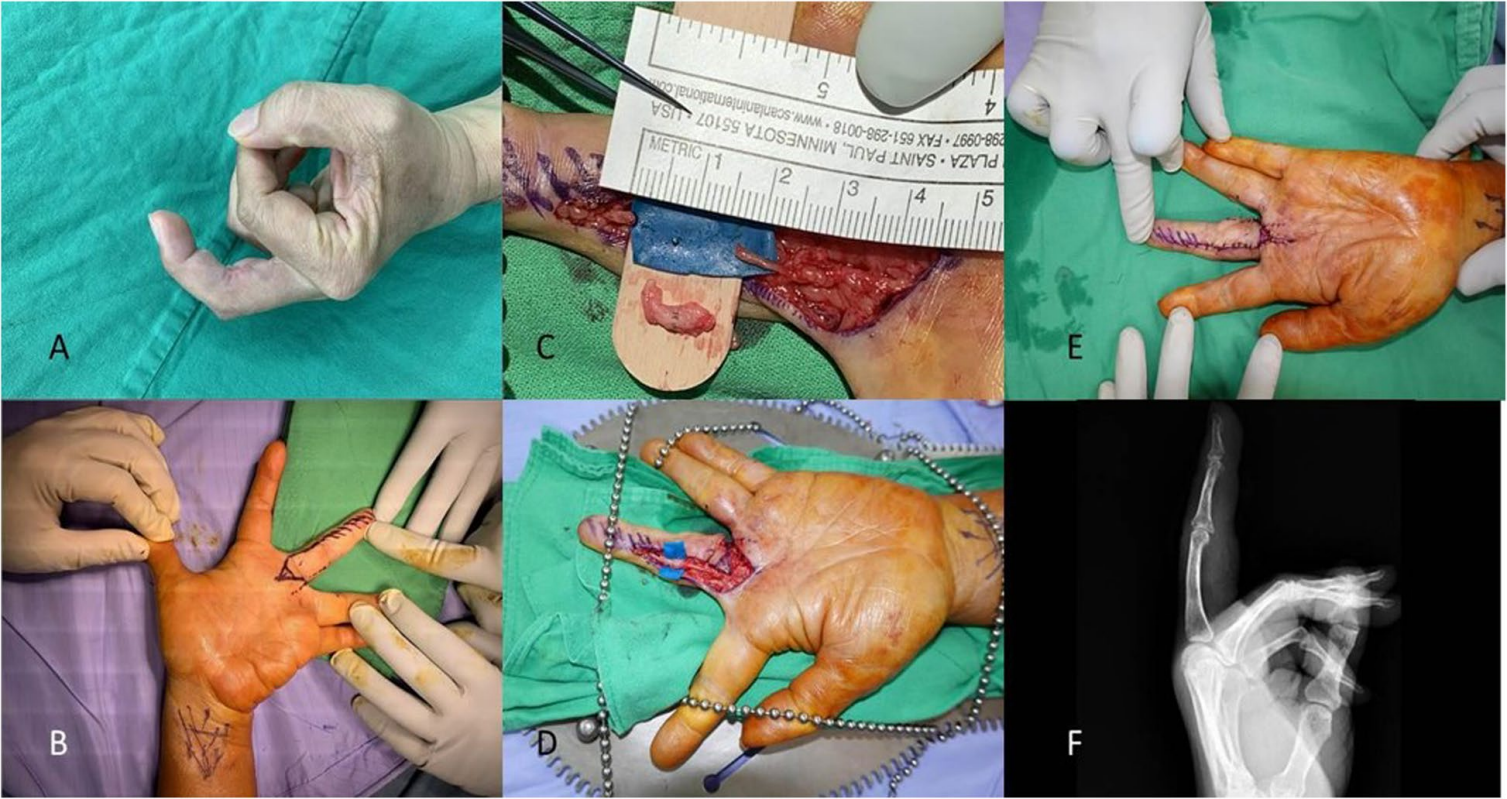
A. Flexion contracture of the left third PIP joint is noted with a 45° extension lag. B. The tip of the advanced V–Y flap is designed 27 mm proximal to the digital base. C. Excision of neuroma is performed. D. Nerve repair is done with sural nerve graft harvested from the right leg. F. Passive full extension of the PIP joint is noted intraoperatively. E. Postoperative plain film showing nearly full active extension of the left third PIP joint
